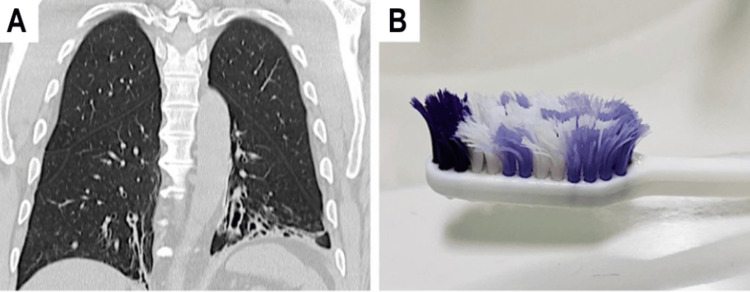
Chronic reinfection in a patient with primary ciliary dyskinesia (PCD) due to colonized toothbrush with Pseudomonas aeruginosa. (A): Coronal view of a chest CT scan showing bibasilar bronchiectasis in a 53-year-old male with PCD homozygous for the RSPH4A (c.921+3_921+6del (intronic)) founder mutation. This patient with PCD is colonized with mucoid *P. aeruginosa*, and regardless of aggressive therapeutic approaches patient continued with recurrent sinopulmonary exacerbations. (B)The toothbrush of the same patient with PCD was used in the past two months as part of his oral hygiene routine. Bacterial cultures were positive for the presence of *P. aeruginosa* on the toothbrush.
